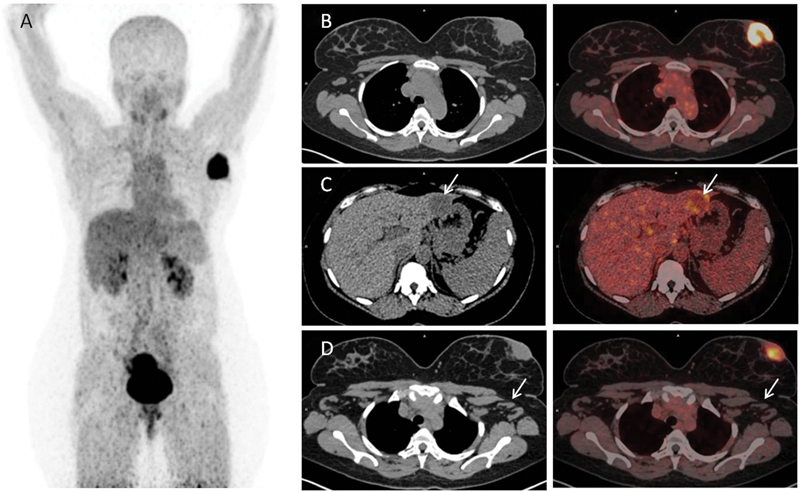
68Ga-FAPI PET/CT : Maximum intensity projection, Axial CT and axial fused PET/CT images (From Left to Right).
